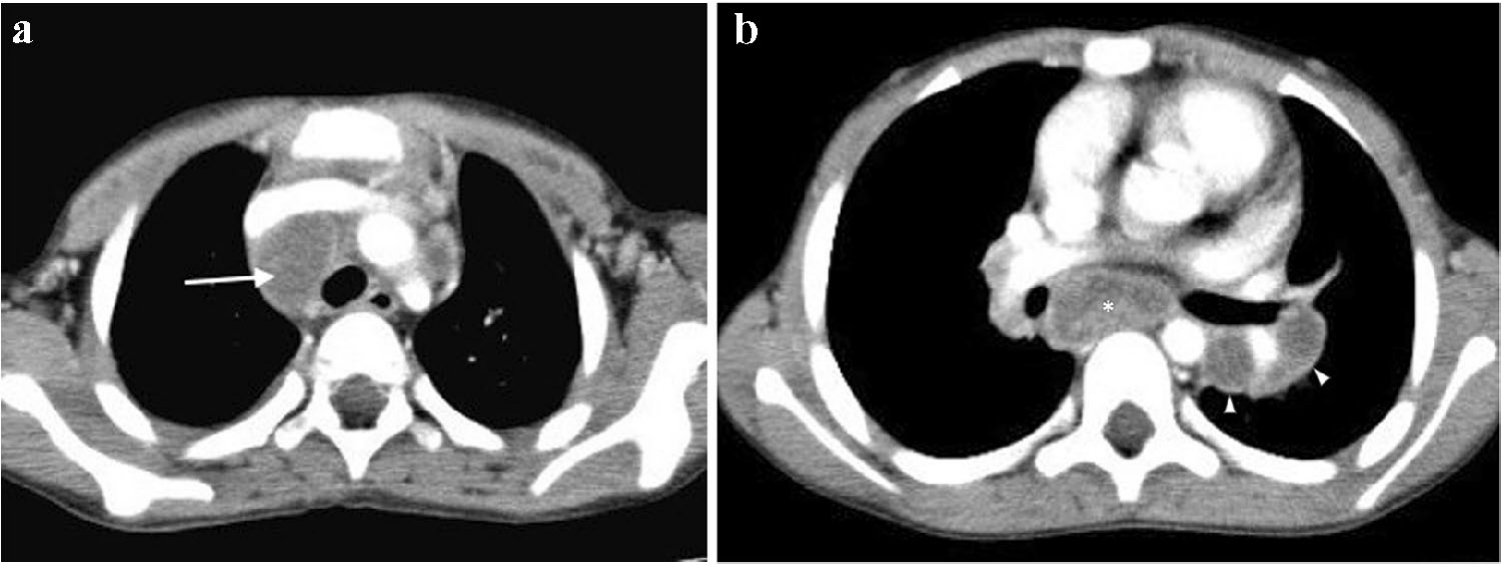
An 8-year-old boy with pulmonary tuberculosis. Axial post-contrast CT (mediastinal window) demonstrates multiple peripherally enhancing necrotic lymph nodes in the mediastinum (*arrow*), left hilum (*arrowheads*) and posterior mediastinum (*asterisk*)
